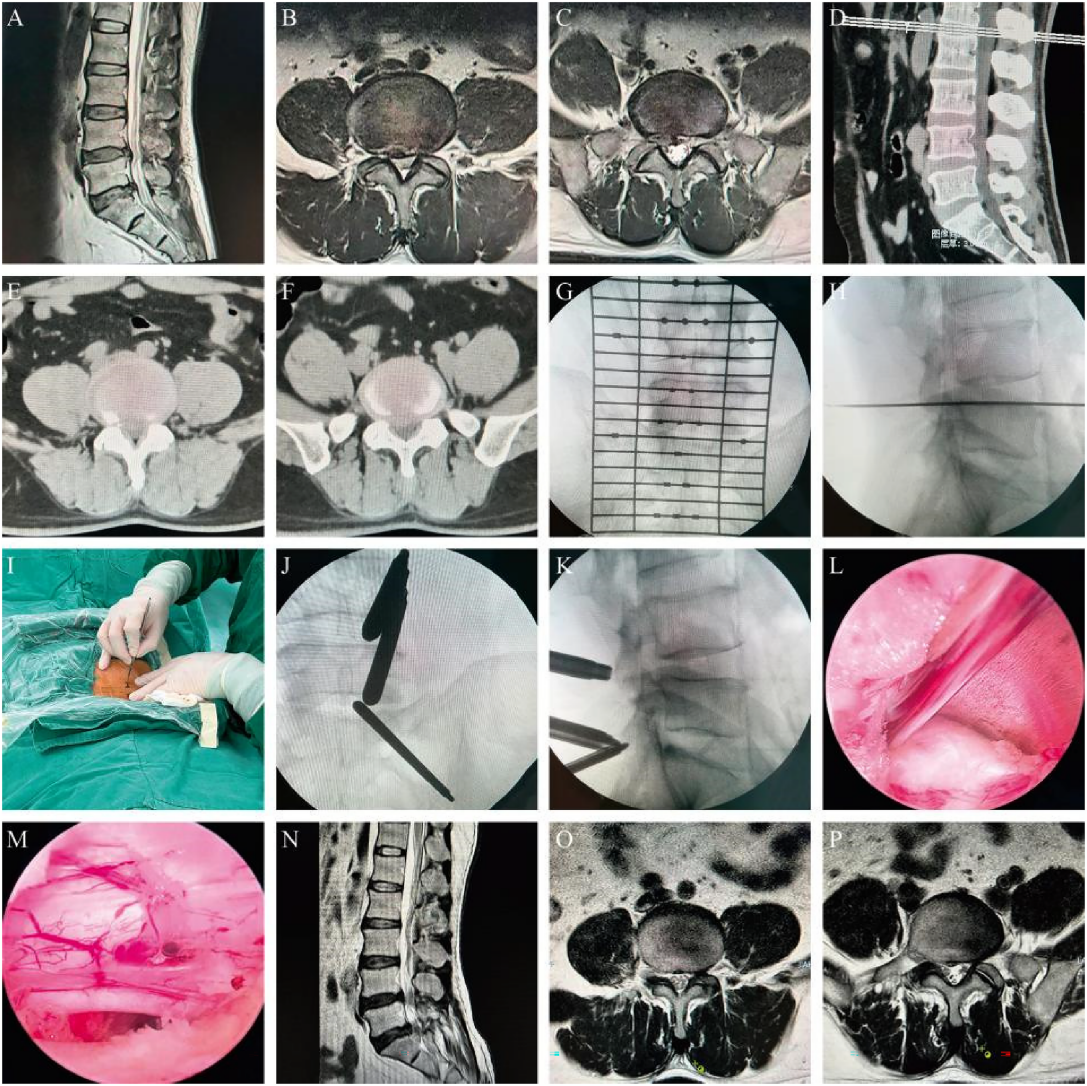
A 65-year-old male patient diagnosed with L4/5 and L5/S1 disc herniation who underwent UBED surgery. (A–F) Preoperative MRI and CT scans; (G,H) preoperative localization; (I) skin incision; (J,K) sheath located at the good position; (L) herniated nucleus pulposus at L4/5 segment; (M) sufficient decompression of the nerve root at the L4/5 segment; and (N–P) postoperative MRI scans showing satisfactory decompression.
